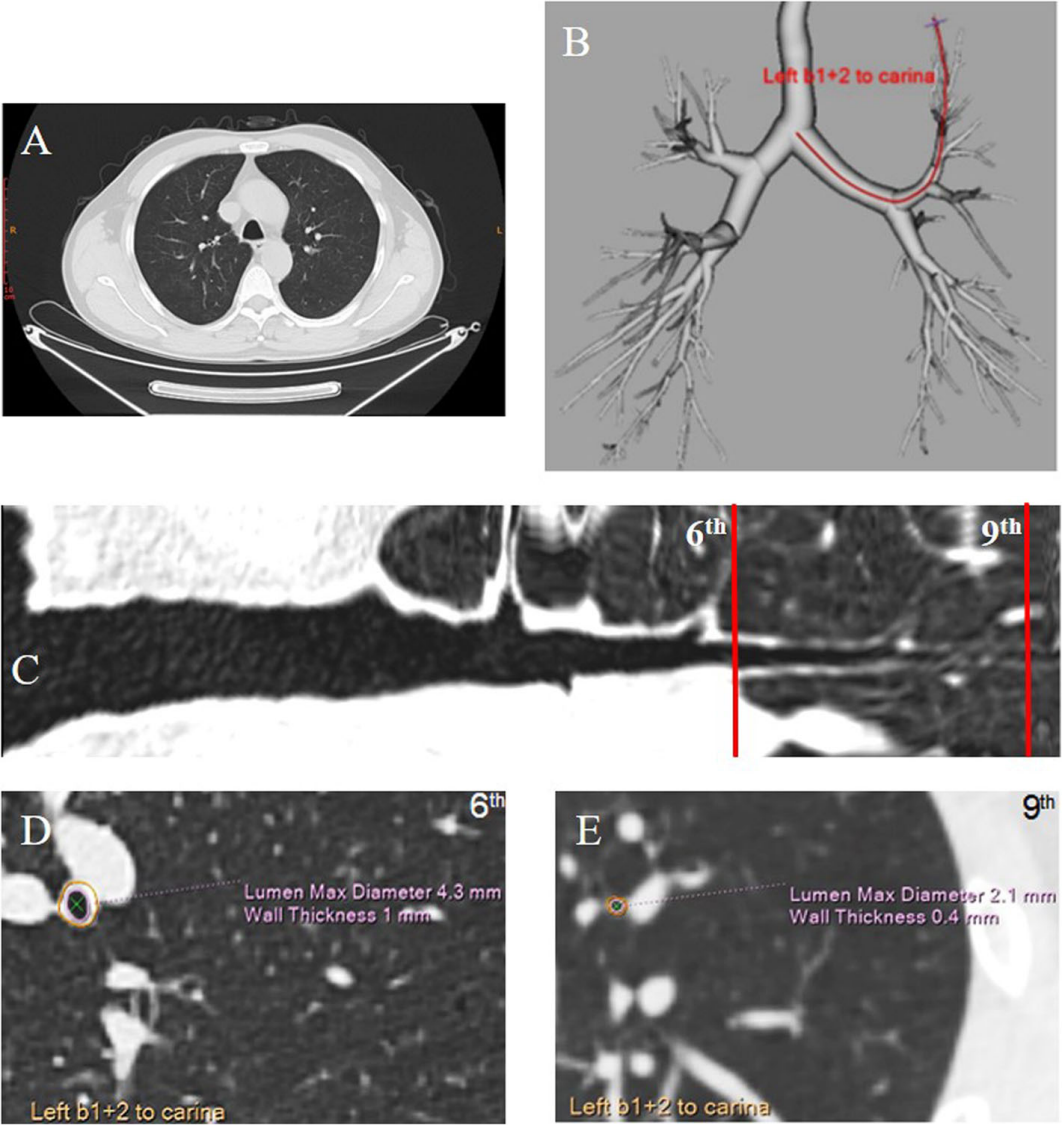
Reconstruction of tracheobronchial tree and measurement of airway dimensions. One axial image of chest CT scan (a) and reconstructed image of tracheobronchial tree (b) for a study subject were presented with the candidate airway for measurement highlighted by the red line. The airway straightened was shown in C with the measurement spots for the 6th and 9th generations labeled by the red lines. The cross-sectional images of airways for dimensional measurement were presented in D (6th generation) and E (9th generation) with lumen diameter and wall thickness labeled
